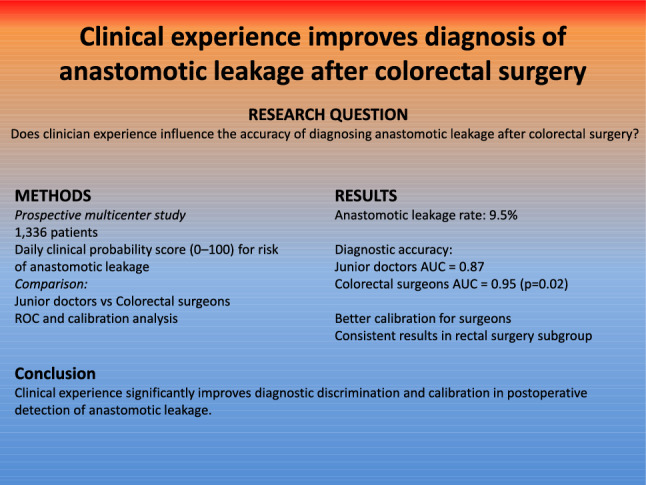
Clinical assessments remain central to medical and surgical decision-making. Despite advances in diagnostic algorithms and the exponential rise in imaging, a clinician’s judgment continues to guide care and relies on a physician’s experience [1, 2]. However, the role of clinical experience in the clinical assessment remains debated. While some evidence suggests that seniority does not necessarily improve diagnostic accuracy of physical examination in acute care settings, other studies have shown the opposite [3, 4]. For instance, physicians with ten or fewer years of experience have been shown to request significantly more inappropriate MRI scans for shoulder, knee, and back pain compared to their more experienced colleagues [3, 4]. At the same time, concerns have been raised that traditional clinical skills are deteriorating in modern medicine, an alarming trend that may compromise patient care [5]. Whether these findings extend to surgical care remains unknown. Yet, the impact of clinical experience may be particularly relevant in high-risk postoperative settings, where subtle clinical changes can signal life-threatening complications. Anastomotic leakage after colorectal surgery provides a valuable model to explore the role of clinical experience, as it is a potentially life-threatening complication whose timely recognition depends heavily on the clinician’s bedside assessment [6–10]. Early diagnosis is essential, yet challenging, as clinical signs are often subtle or nonspecific. While structured tools such as the Dutch Leakage (DULK) score aim to standardize evaluation, bedside assessments remain subjective and are likely influenced by a clinician’s level of experience [11, 12]. Surprisingly, it remains unclear whether the clinical suspicion of a junior doctor carries the same diagnostic value as that of a senior physician in postoperative care. Clarifying this relationship may enable more targeted supervision, reduce unnecessary abdominal CT scans, and support the integration of experience-weighted assessments into structured postoperative care pathways.
